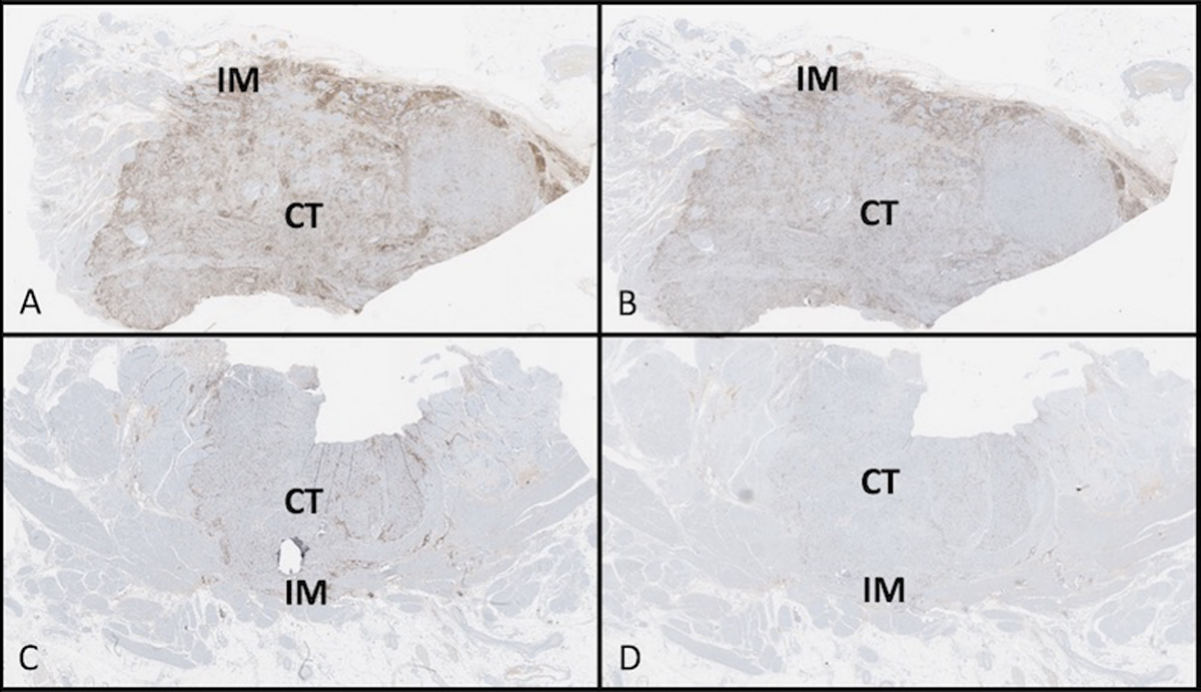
High vs. low density and locations of CD8^+^. (A) High concentration CD3^+^ in CT and IM. (B) High concentration CD8^+^ in CT and IM. (C) Low concentration CD3^+^ in CT and IM. (D) Low concentration CD8^+^ in CT and IM.
